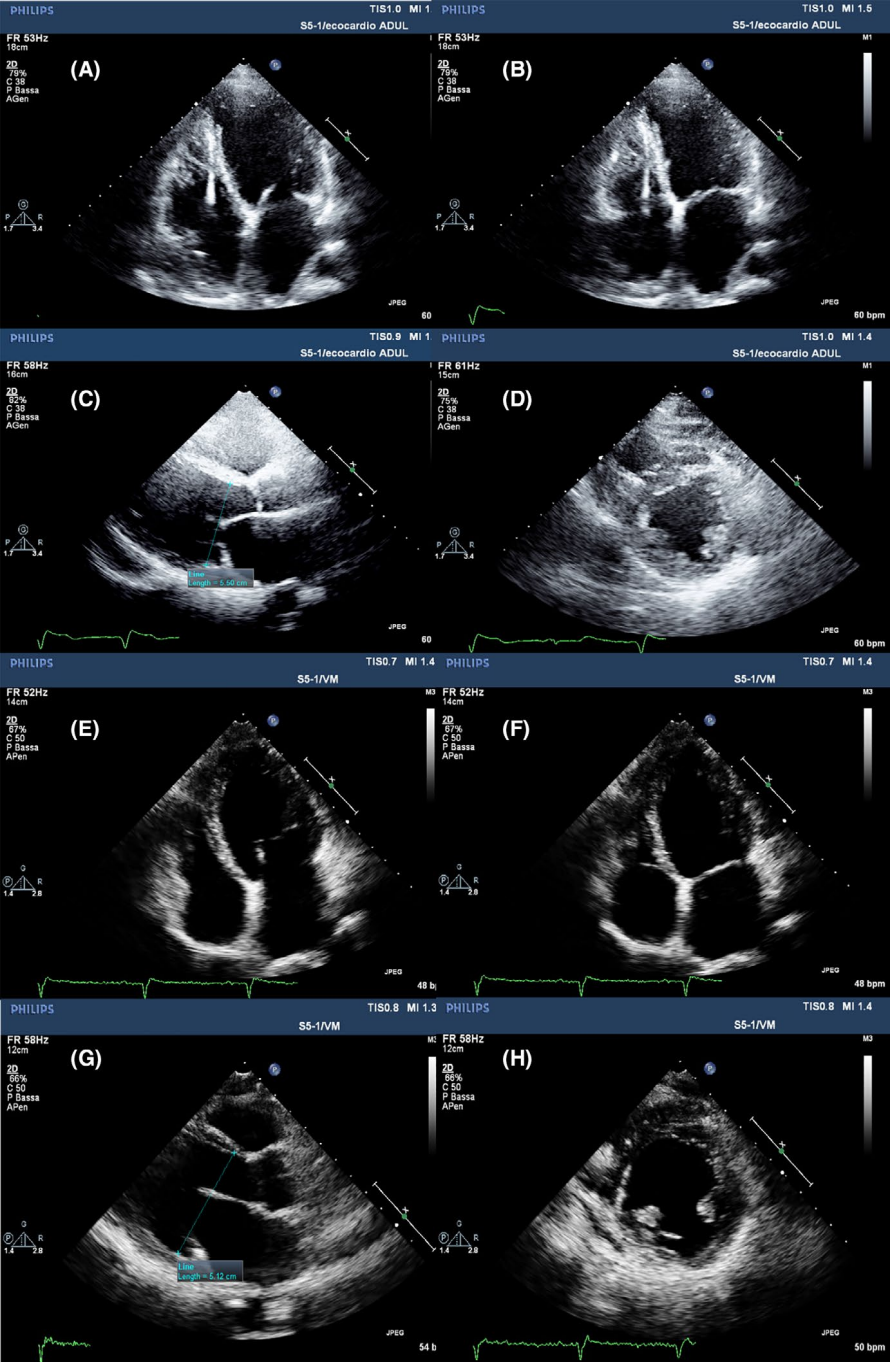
Cardiac phenotype evaluated by Echocardiographic imaging in LMNA mutants’ carriers. The upper half of the image shows LMNA DCM phenotype: (A) and (B) panels show apical four chamber (4C) views with chamber dilatation (LVEDVi 82 ml/m^2^ and LVESVi 52 ml/m^2^) and reduced left ventricular systolic function (LVEF 35%) due to global hypocontractility. (C) PLAX view: LVEDD (55 mm). (D) PSAX view: mid‐LV at the level of papillary muscles. The lower part (panels E–H) of the picture shows a hypokinetic non‐dilated LMNA cardiomyopathy characterized by normal ventricular volumes both in end‐diastole (E) (LVEDVi 64 ml/m^2^) and end‐systole (F) (LVESVi 38.8 ml/m^2^) with moderate systolic left ventricular dysfunction (LVEF 36%). (G) In PLAX view: LVEDD (51 mm). (H) PSAX view: mid‐LV at the level of papillary muscles. DCM, dilated cardiomyopathy; LVEDD, left ventricular end‐diastolic diameter; LVEDV, left ventricular end‐diastolic volume index; LVEF, left ventricular ejection fraction; LVESVi, left ventricular end‐systolic volume index; PLAX view, parasternal long‐axis view; PSAX, parasternal short‐axis view
